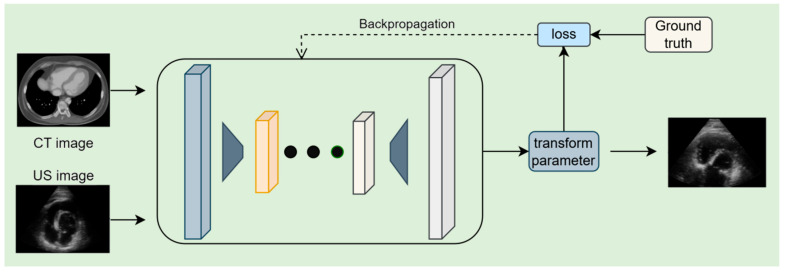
Cardiac CT–ultrasound registration model framework based on supervised learning for preoperative surgical planning.
